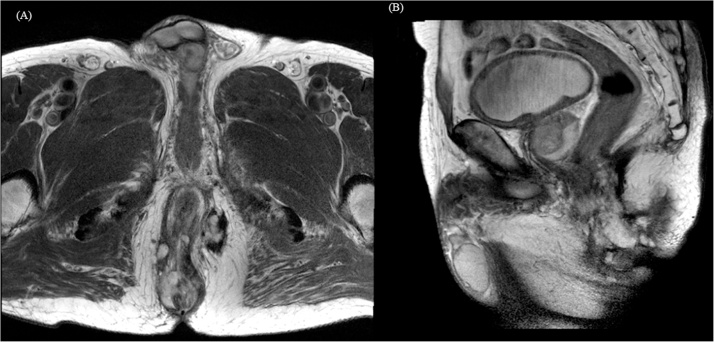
Magnetic resonance image (MRI) findings. MRI showed perianal fistula and associated perianal multifocal abscess cavity. There was no evidence of lymphadenopathy in perirectal space, both internal iliac vessel area. (a) T2 weighted Magnetic resonance imaging. Axial view. (b) T2 weighted Magnetic resonance imaging. Sagittal view.
